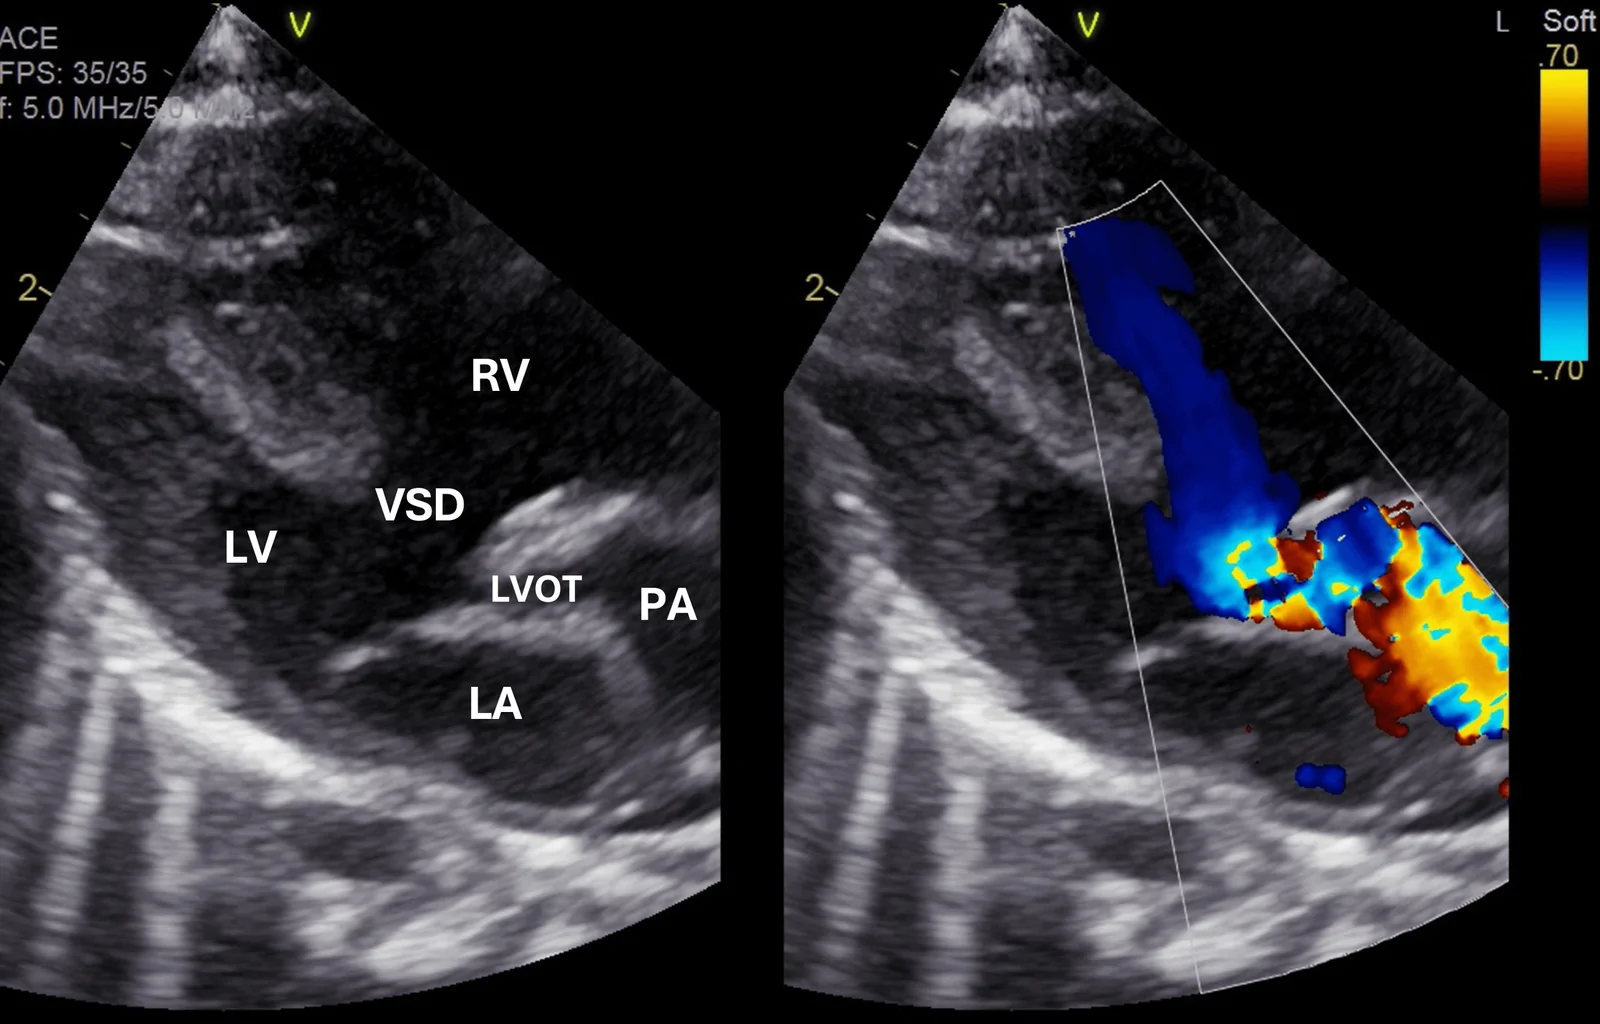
Preoperative echocardiogram. (Left) Two-dimensional image showing the right ventricle, left ventricle, left atrium, pulmonary artery, and the perimembranous ventricular septal defect. (Right) Corresponding color-flow Doppler image demonstrating turbulent flow across the ventricular septal defect and the left ventricular outflow tract. LA, left atrium; LV, left ventricle; LVOT, left ventricular outflow tract; PA, pulmonary artery; RV, right ventricle; VSD, ventricular septal defect.
